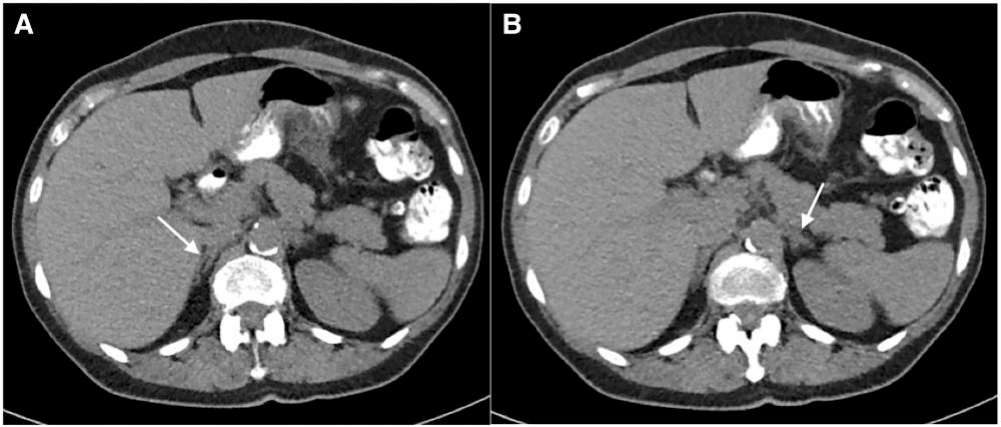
(A) Patient A's CT abdomen and pelvis noncontrast showing normal right adrenal gland. (B) Patient A's CT abdomen and pelvis noncontrast showing normal left adrenal gland. Abbreviation: CT, computed tomography.
